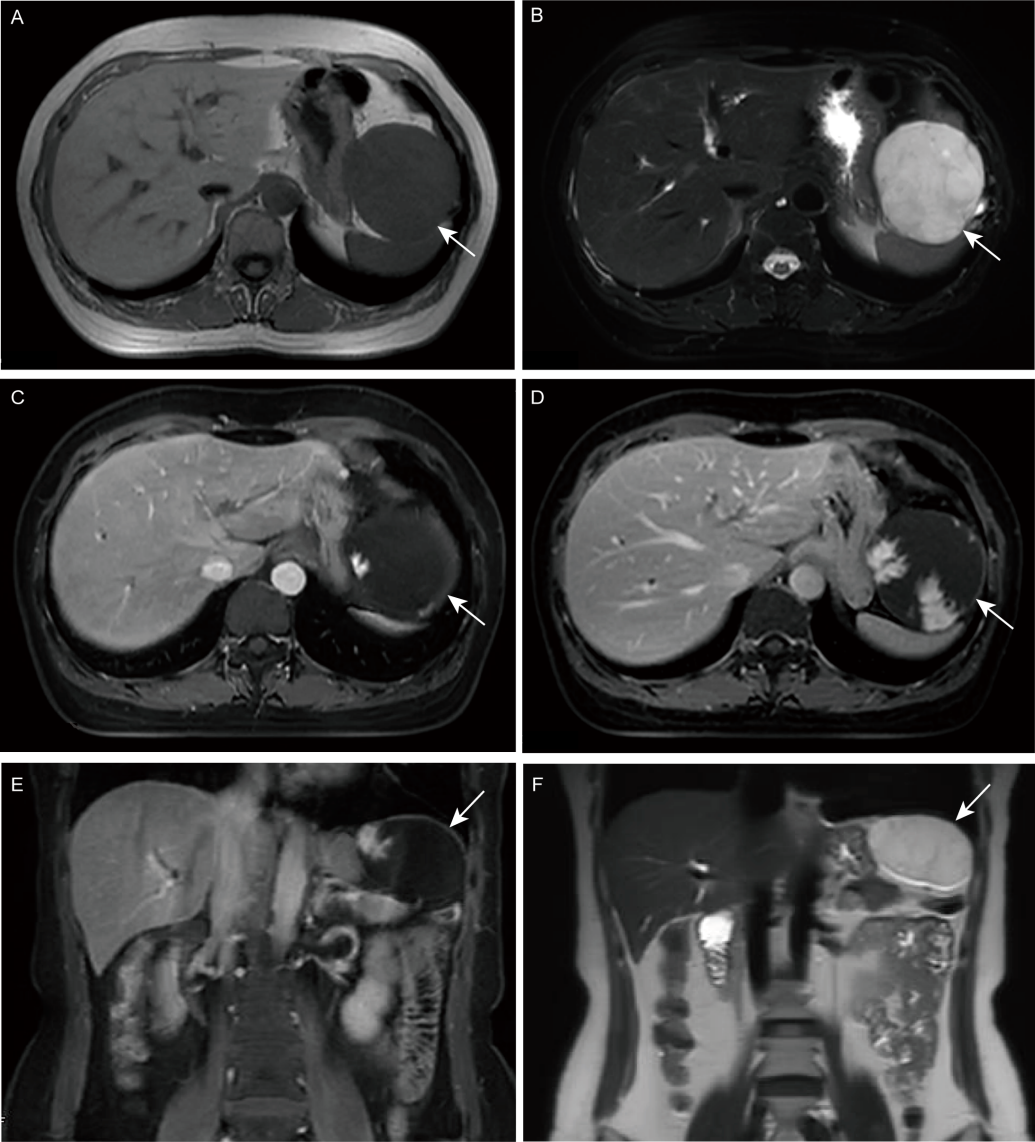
Magnetic resonance imaging (MRI). (A, E) T1-weighted image (T1WI); (B, F) T2-weighted image (T2WI); (C) Enhanced arterial phase; (D) Enhanced portal phase.
